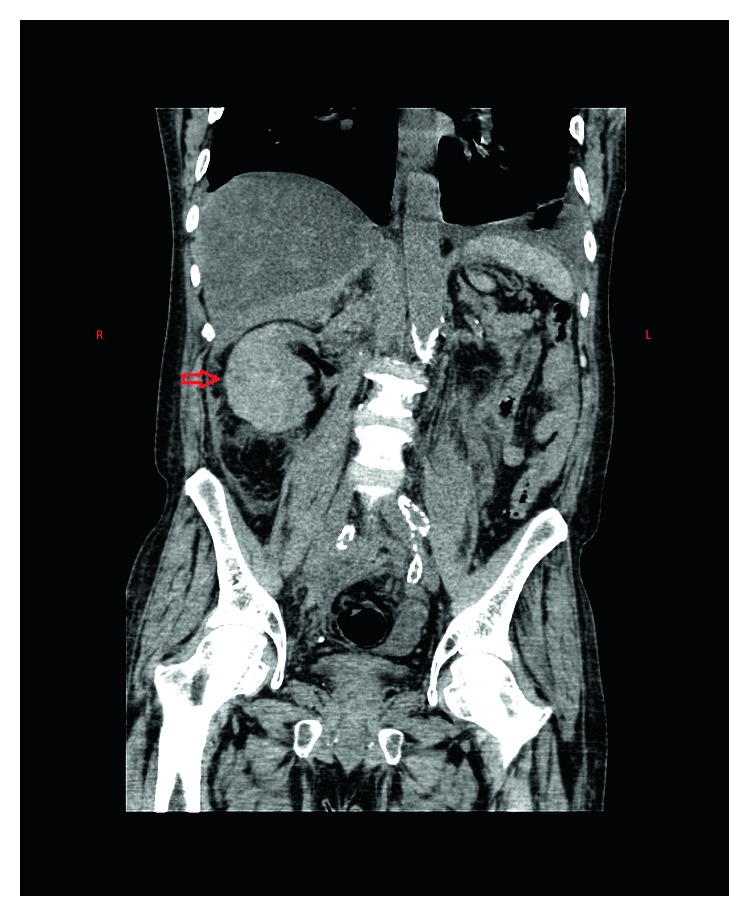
Coronal section of the noncontrast CT scan of the abdomen showing an enlarged right kidney (marked in red arrow) suggesting a potential collection with or outside the renal capsule.
